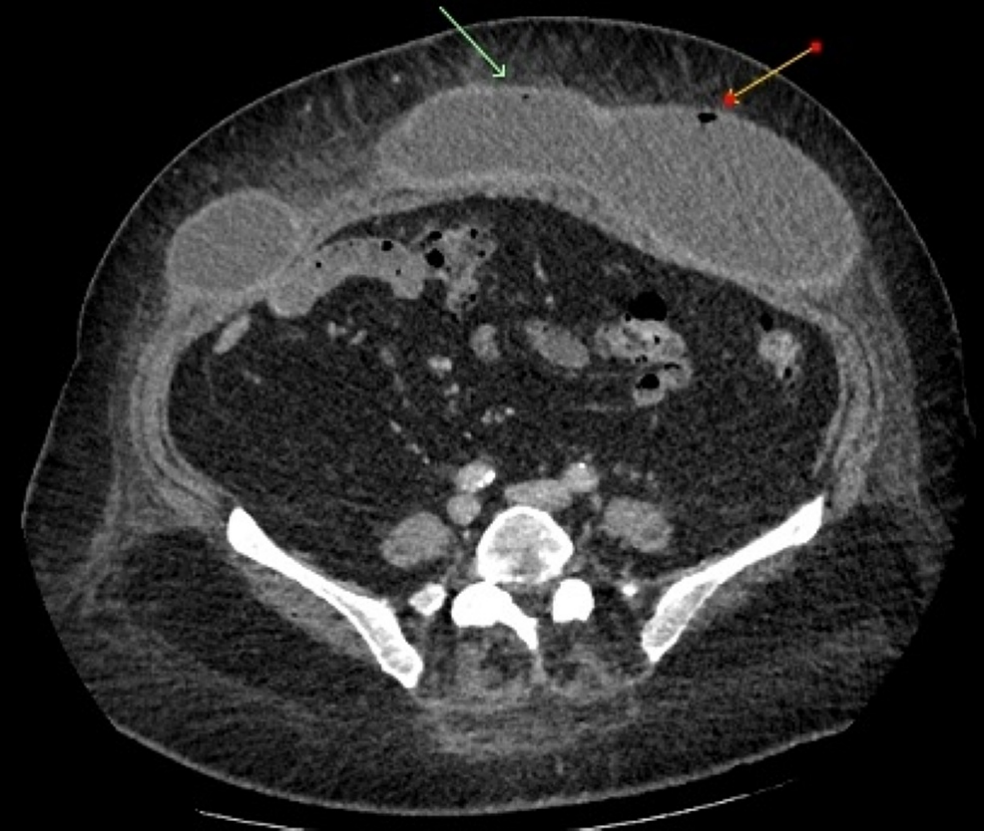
CT abdomen post ERCP (axial slice). Arrows pointing to gas locules within seroma.
